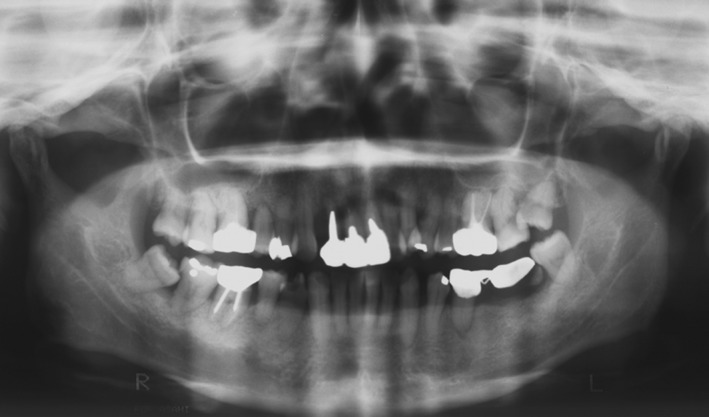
Panorama radiograph at the first visit.
